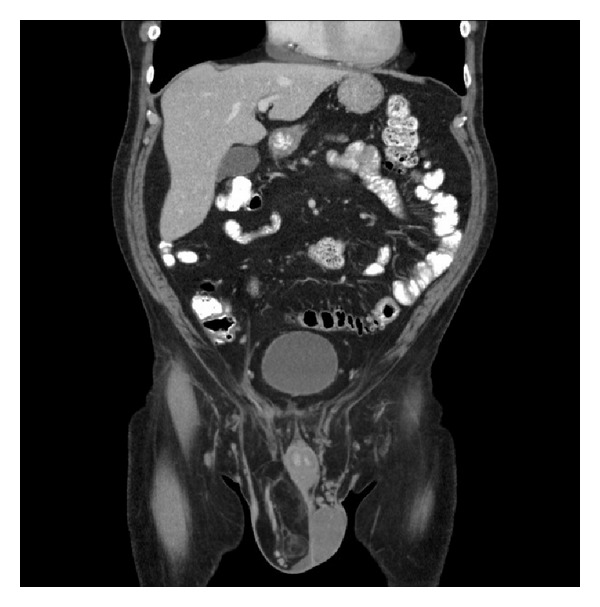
CT abdomen/pelvis read as a large fat-containing inguinal hernia present on the right side extending into the scrotal sac. No bowel loops are contained but there is a significant amount of omentum and fat present.
